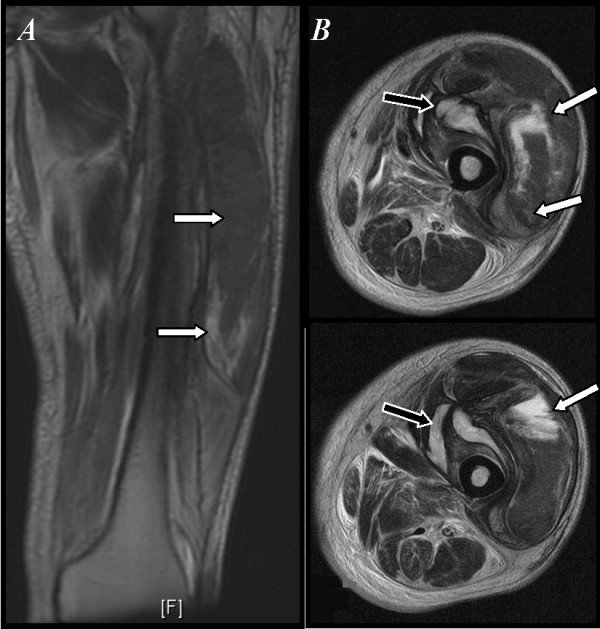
Magnetic resonance imaging of the left thigh. A) Coronal slice on T-2 sequence: hyperintense image of the quadriceps muscle in relation to inflammatory changes and necrosis (arrows). B) Axial slice on T-2 sequence: hyperintense image of the quadriceps muscle (white arrow), there is a fluid collection lateral to the vascular bundle (black arrow).
